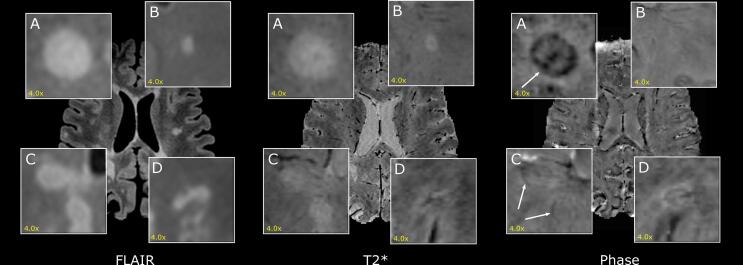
Example of MS lesions on 3D FLAIR (left), 3D-EPI magnitude (center) and phase (right) images. A) and B) are clear examples of lesions with presence (rim+) and absence (rim-) of a paramagnetic rim, respectively. In C) there are two more subtle rim+ lesions. D) is an example of a rim- lesion that has a rim+ like intensity artefact.
